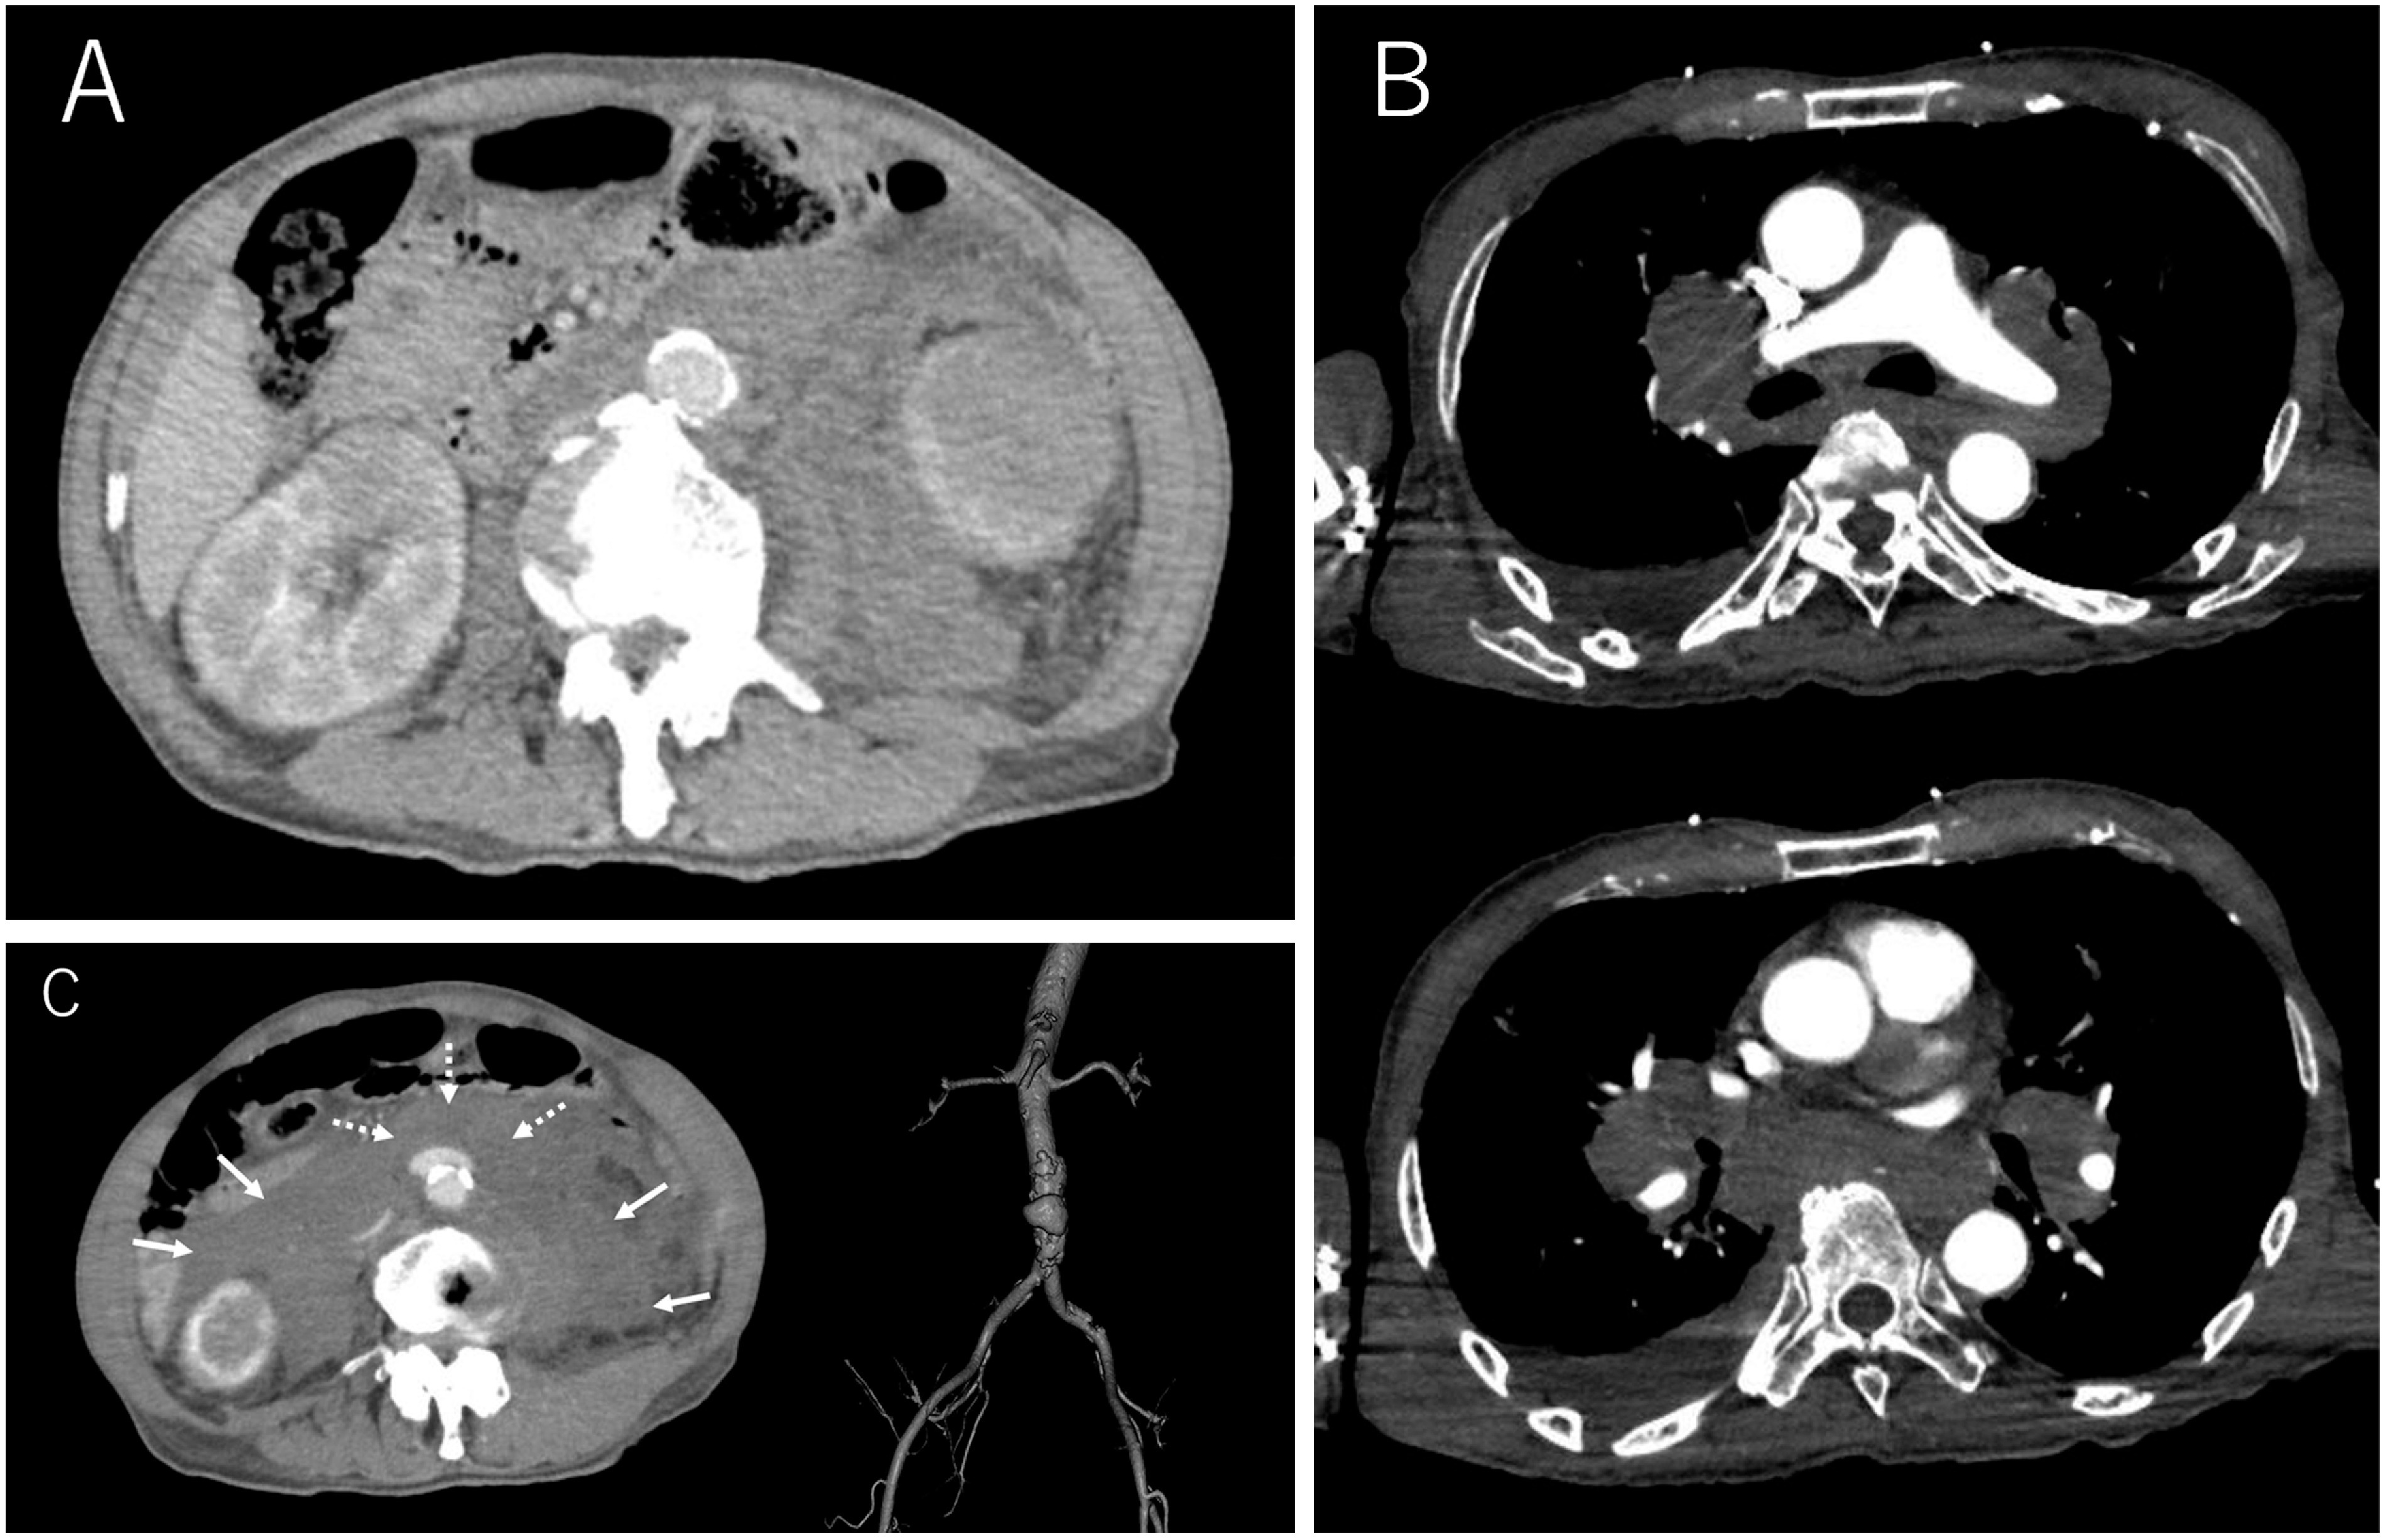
Fig. 1 Preoperative contrast enhanced computed tomography (CT) scan.
